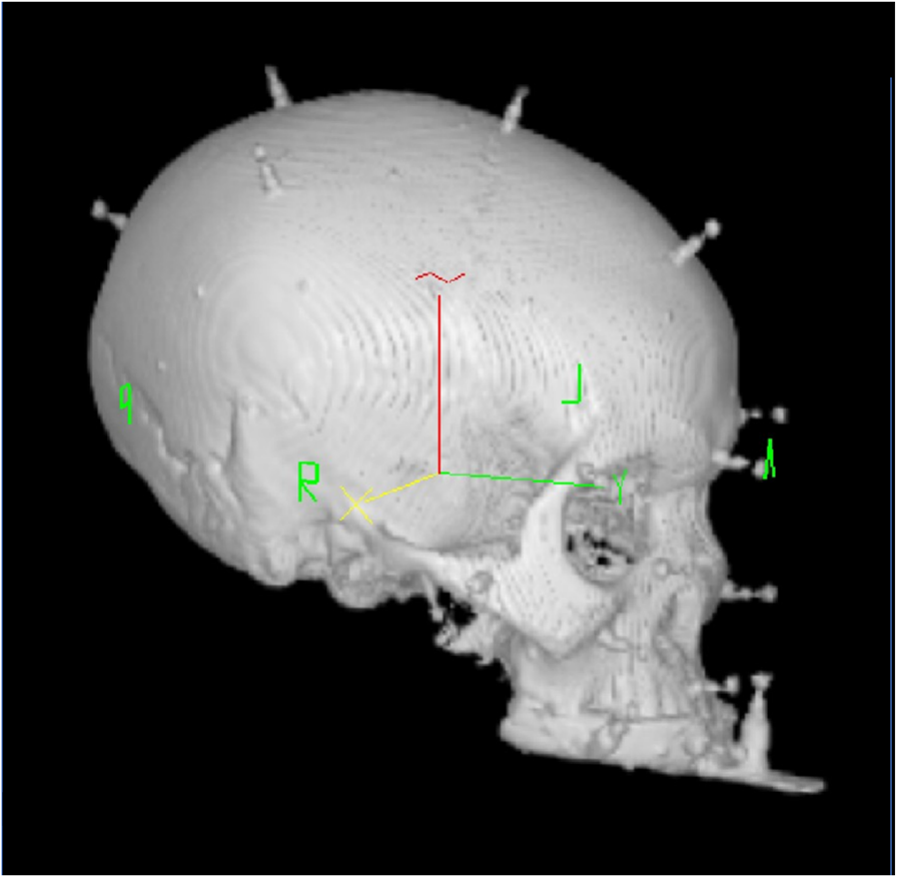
Three-dimensional reconstructed computed tomography of the skull with an inserted maxillary splint and twenty additionally inserted bone markers for metric accuracy testing
